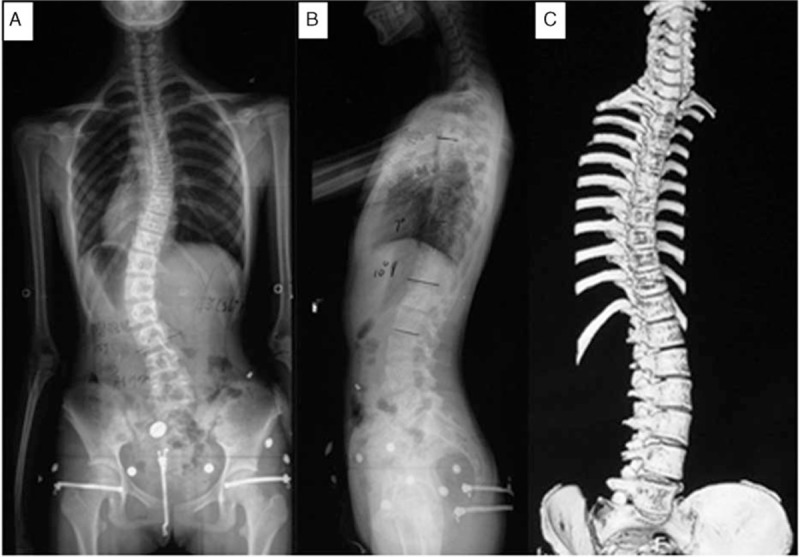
A 16-year-old female patient with adolescent idiopathic scoliosis. Preoperative thoracic and lumbar curve were 36° and 40°, respectively. Eleven thoracic vertebrae and 6 lumbar vertebrae was found on the full-spine anteroposterior and lateral plain films (A and B) and determined by the 3D-reconstruction preoperatively (C).
